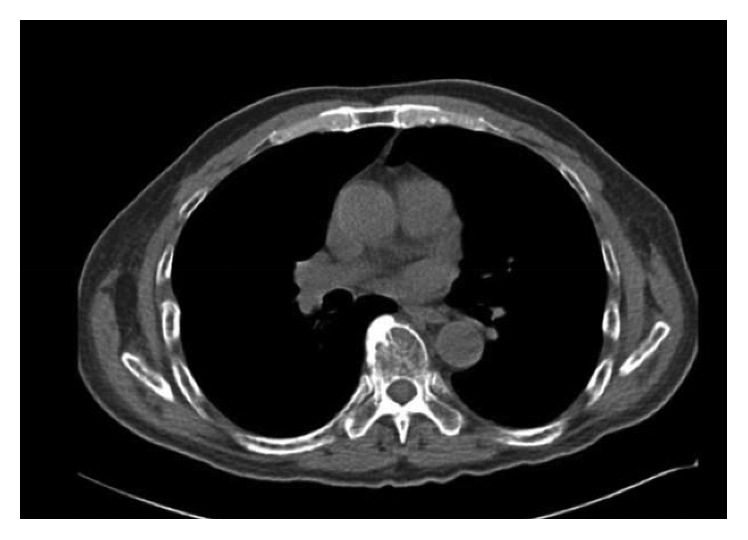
Prior CT chest for comparison.
